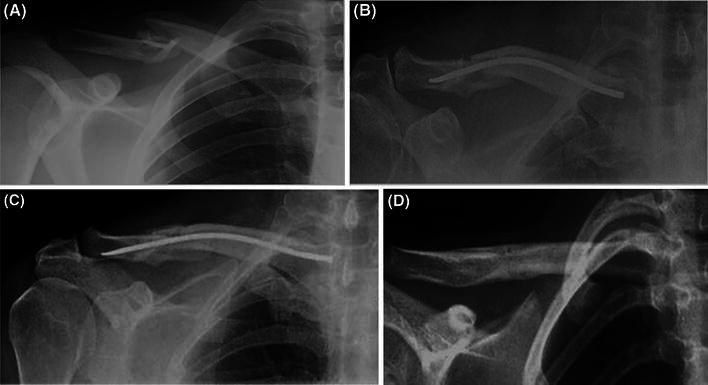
a Preoperative X-ray of 26-year-old male patient showing displaced midshaft clavicle fracture *right side*. b Fracture reduced and fixed with antegrade titanium elastic nail. c Postoperative X-ray at 12 weeks showed fracture uniting well with nail in situ. d Postoperative X-ray showing united fracture (elastic nail removed)
